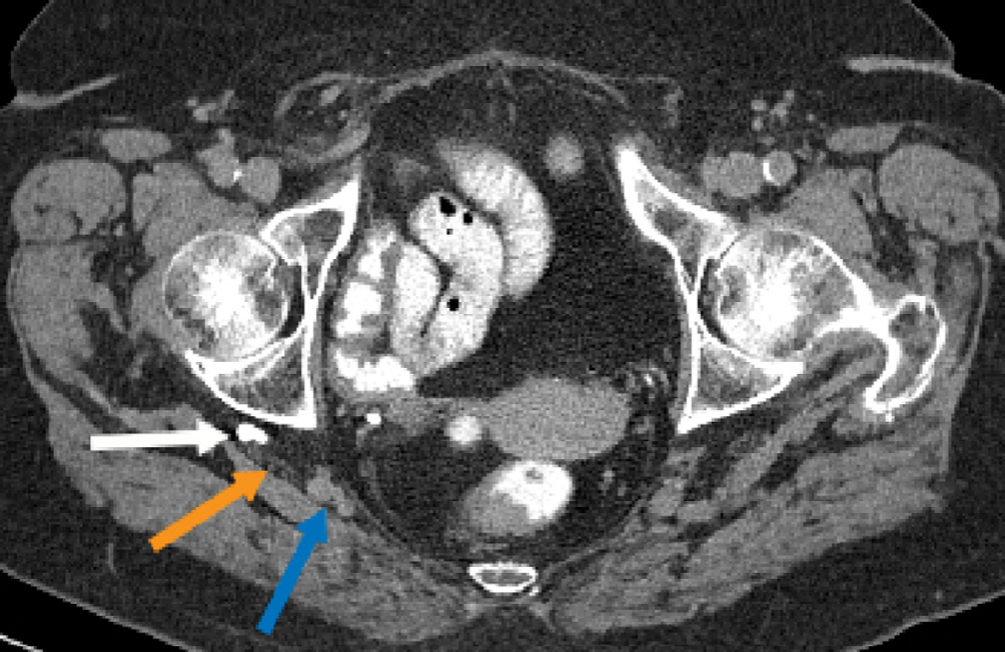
Axial CT image in the delayed phase shows a tubular structure opacified with contrast posterior to the right acetabulum, consistent with a right ureterosciatic hernia (white arrow). This abuts the right sciatic nerve (orange arrow). The inferior gluteal vessels are medial to this (blue arrow) (color version of figure is available online).
